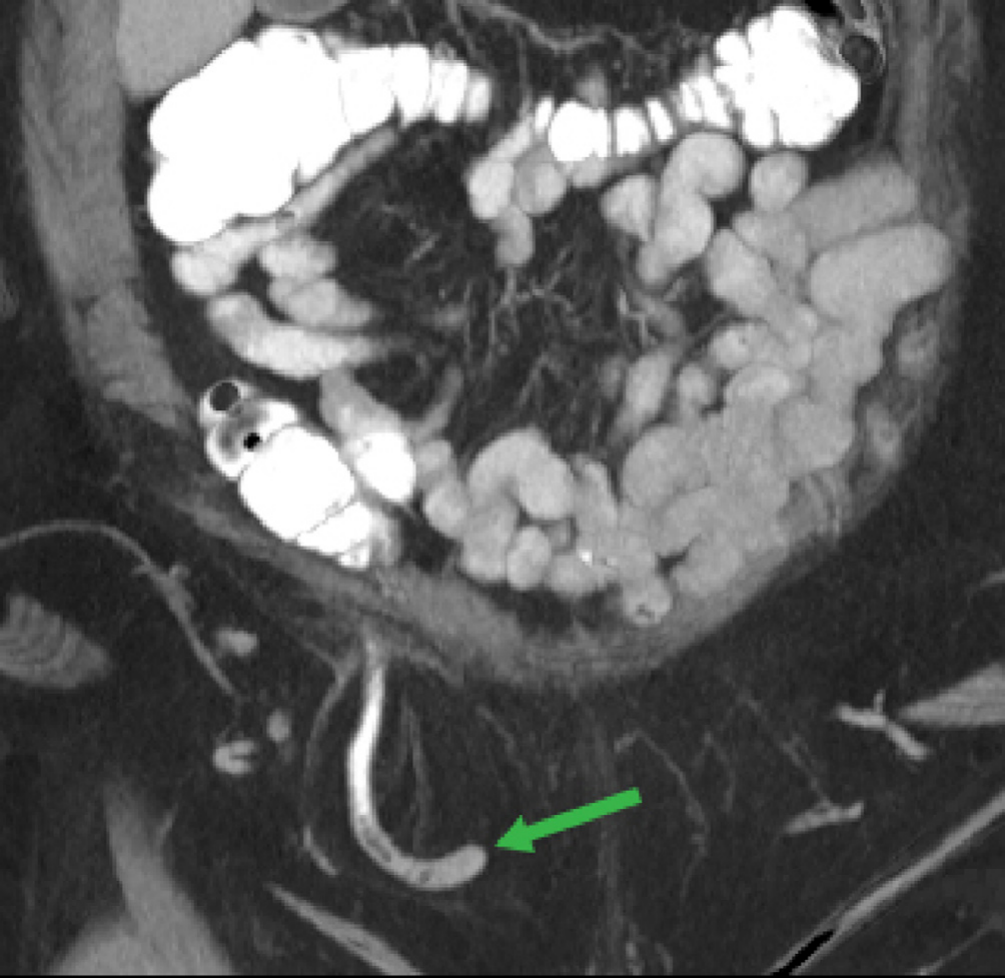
Coronal CT maximum intensity projection demonstrates a right inguinal hernia containing the appendix, consistent with an Amyand hernia (green arrow). Oral contrast is present in the bowel and appendix (color version of figure is available online).
